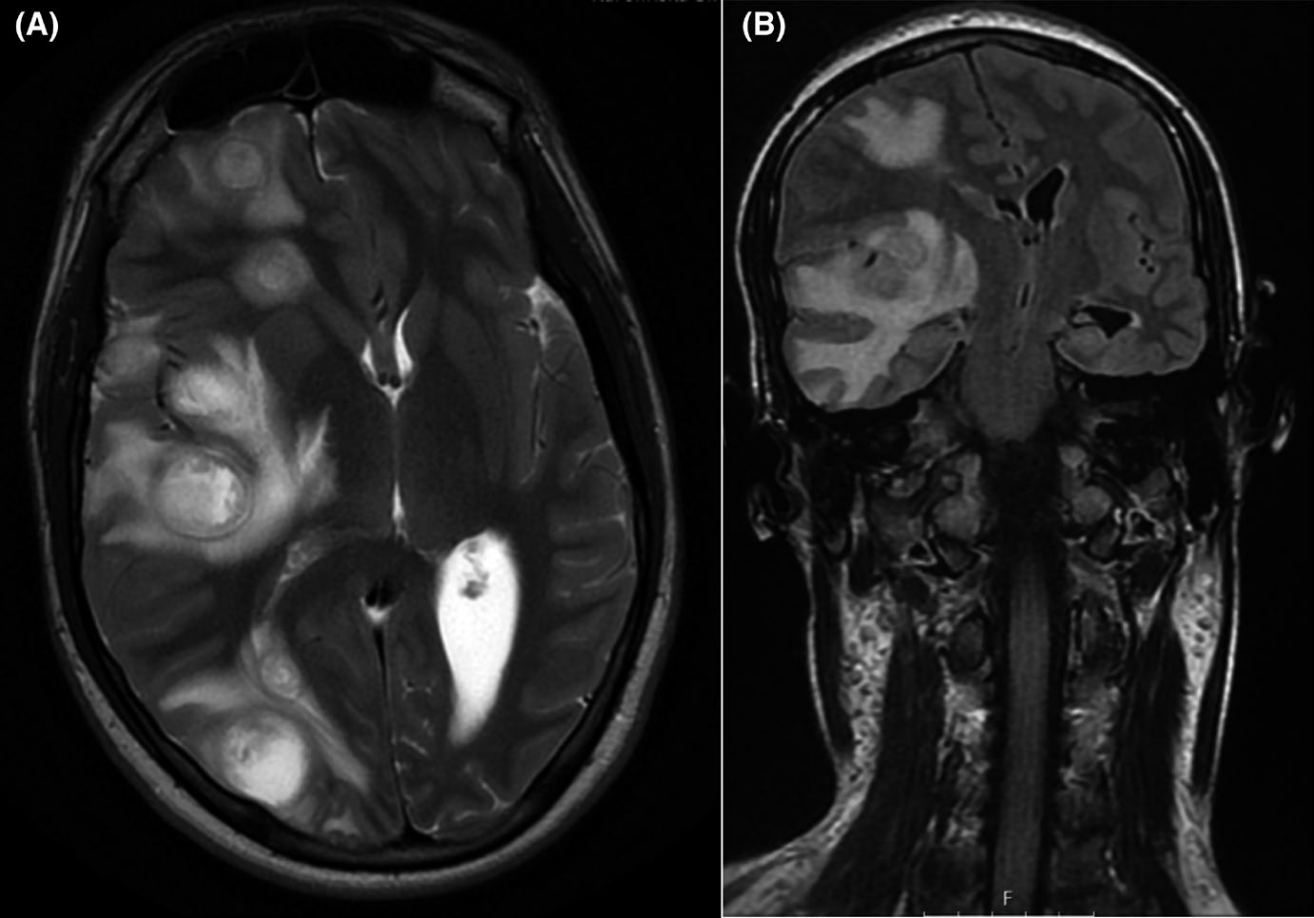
MRI Day two; axial (A) and coronal (B) T2‐weighted image demonstrating increasing edema and mass effect of intracranial abscess lesions
